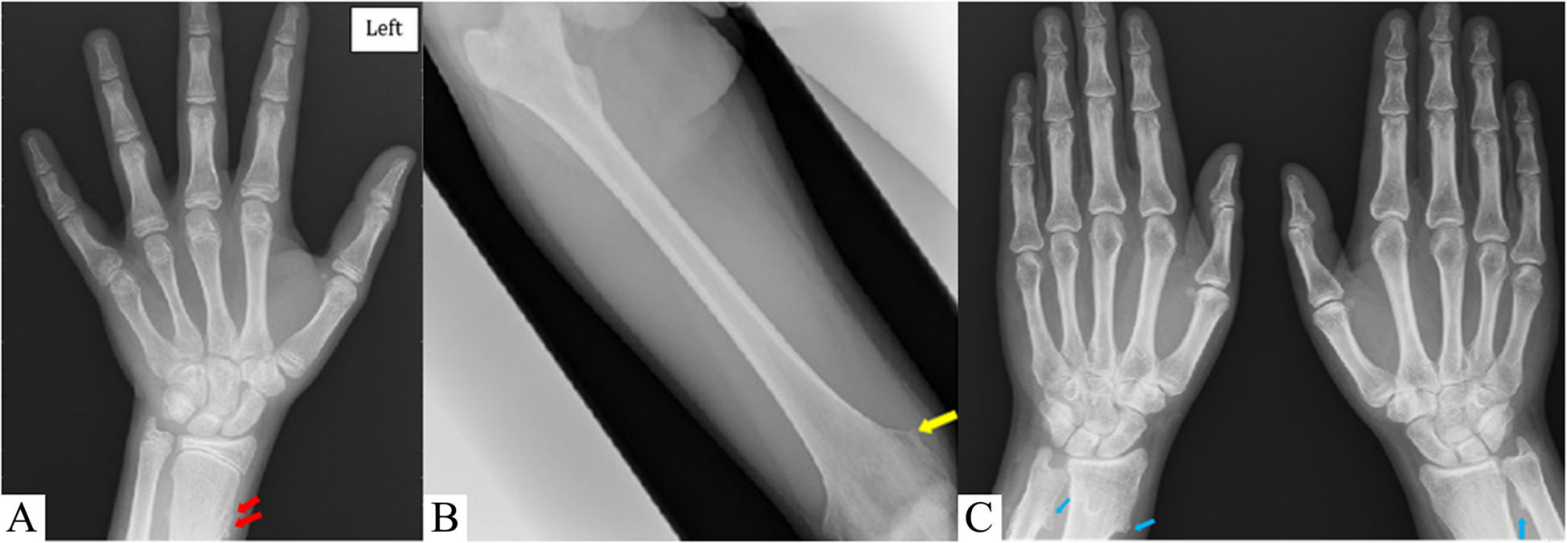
a Left hand X-ray, showing multiple bulky cartilage cap in the distal region of the radius suggesting multiple osteochondromas (red arrow). b Right femur X-ray, showing an uninterrupted flow of cortex and medullary bone from the host bone into an area of new bone proliferation in the medial side of the distal femur (yellow arrow), confirming the hypothesis of multiple osteochondromas. c Bilateral hands X-rays of the girl’s mother, showing multiple osteochondromas (only a few of which outlined by blue arrows)
